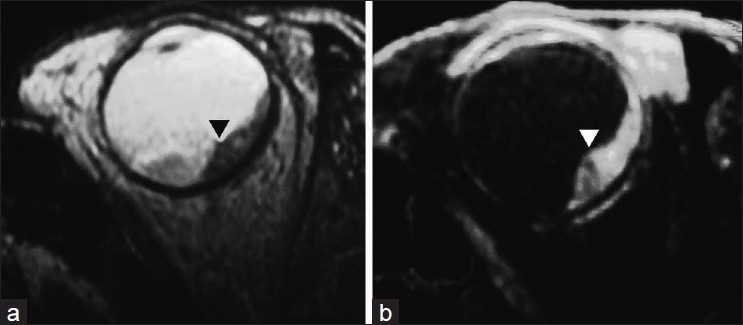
Magnetic resonance imaging shows a plaque-like lesion of uneven thickness along the posterolateral surface of the globe in close relation to the choroid (►) with associated retinal detachment. The lesion is hypointense on T2W study and hyperintense on STIR images
